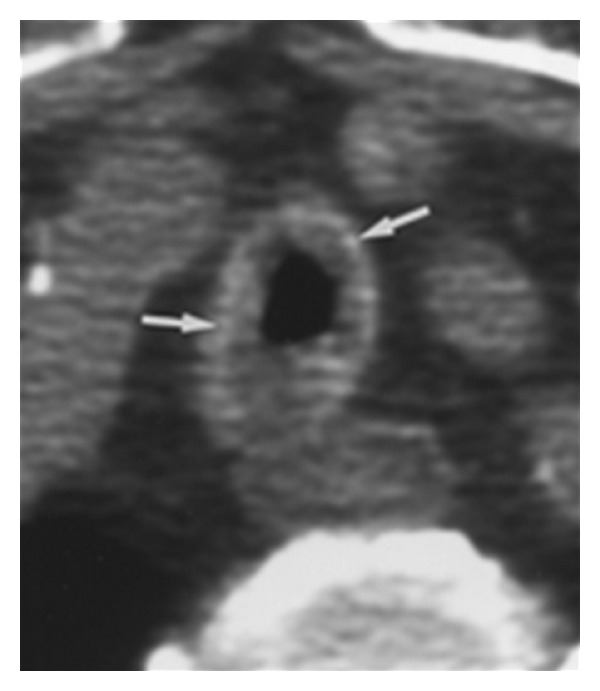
CT scan with amyloid deposits narrowing the tracheal diameter.
